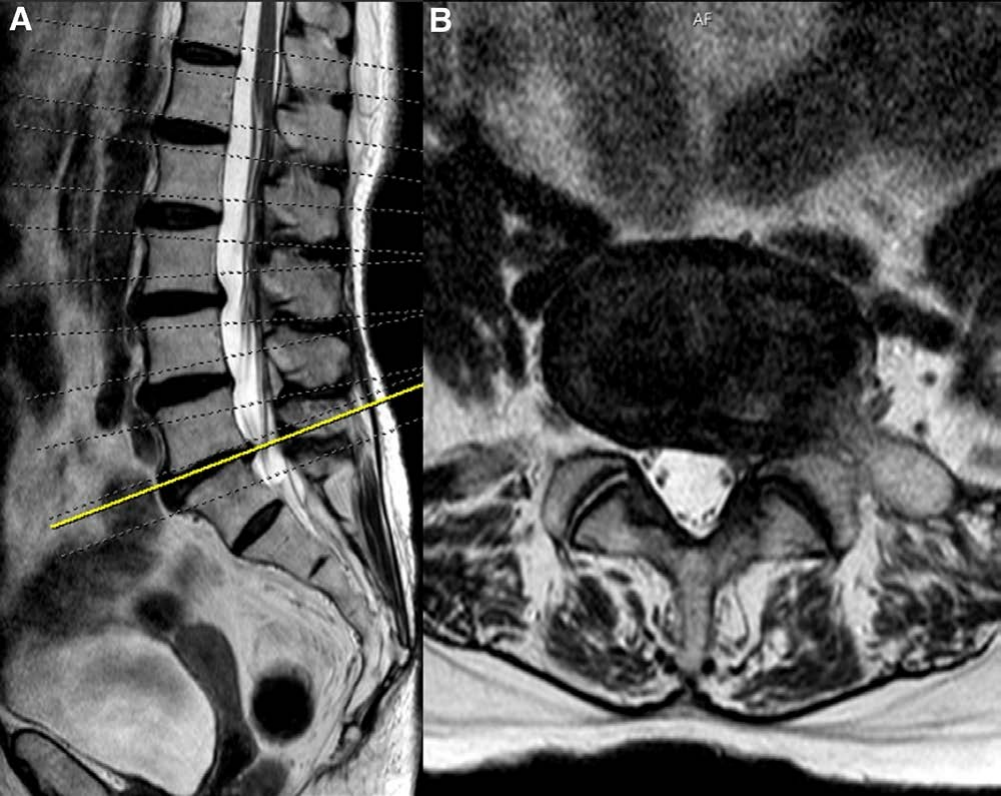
Lumbar spine MRI showing lumbar 5-sacrum 1 foraminal stenosis and disc extrusion ((A) sagittal view and (B) axial view). MRI = magnetic resonance imaging.
